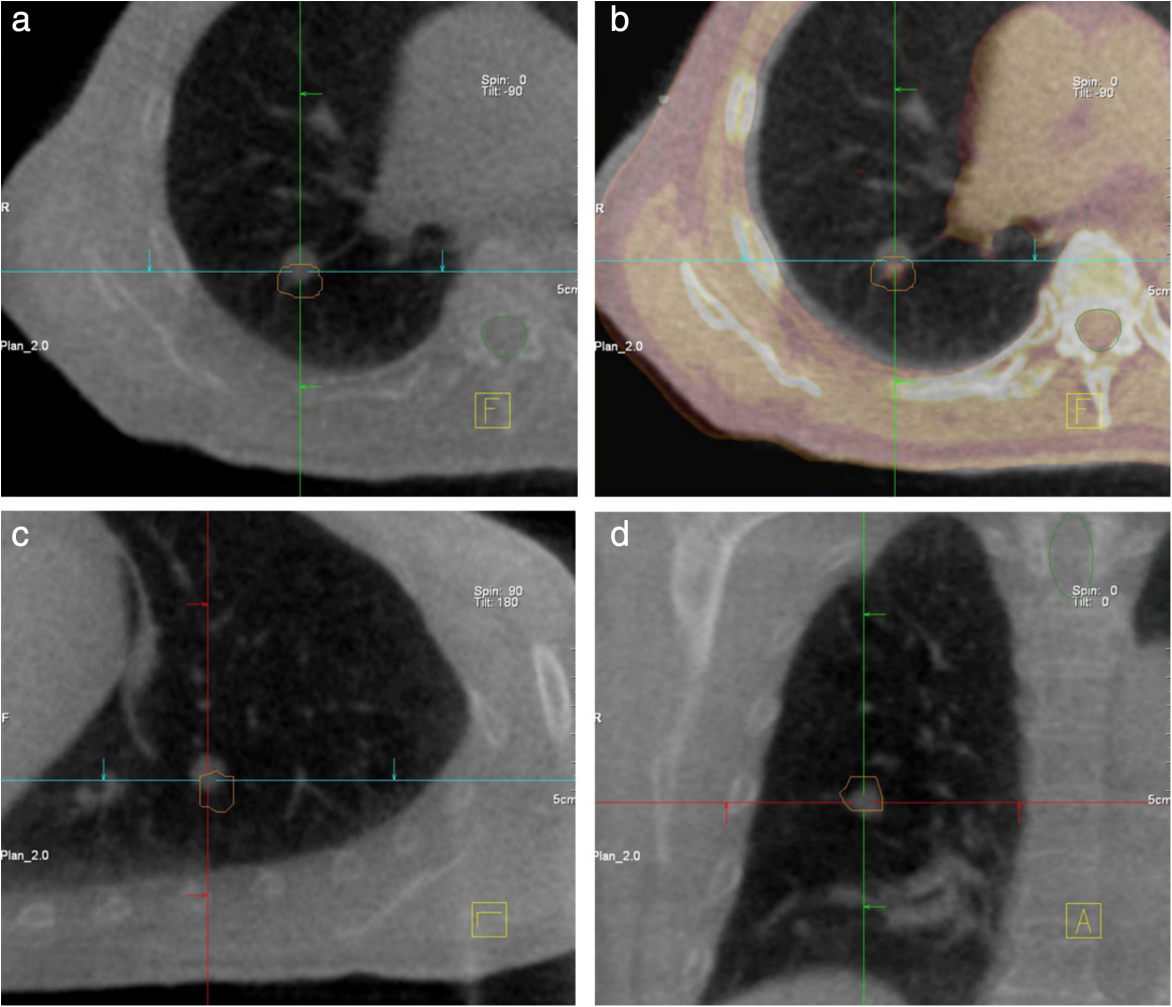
Example of the correction mismatch between the vertebra and tumor matching. There was a considerable mismatch between the cone‐beam computed tomography (CBCT)‐visualized tumor and the internal target volume (ITV, orange color) of the planning CT with a rotational setup error (b), despite accurate vertebra matching. (a and b) Transverse view; (c and d) sagittal (c) and coronal (d) views.
